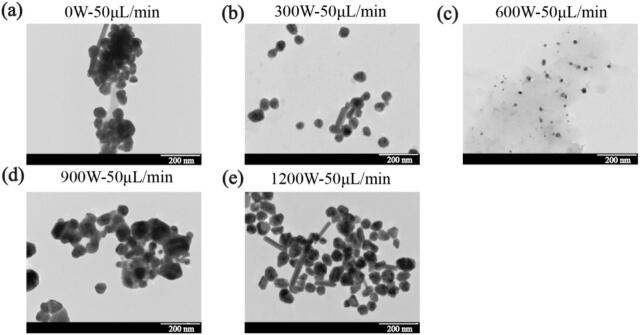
TEM images of AgNPs prepared under different ultrasonic power condition (scale bar = 200 nm): (a) 0 W; (b) 300 W; (c) 600 W; (d) 900 W; (e) 1200 W.
